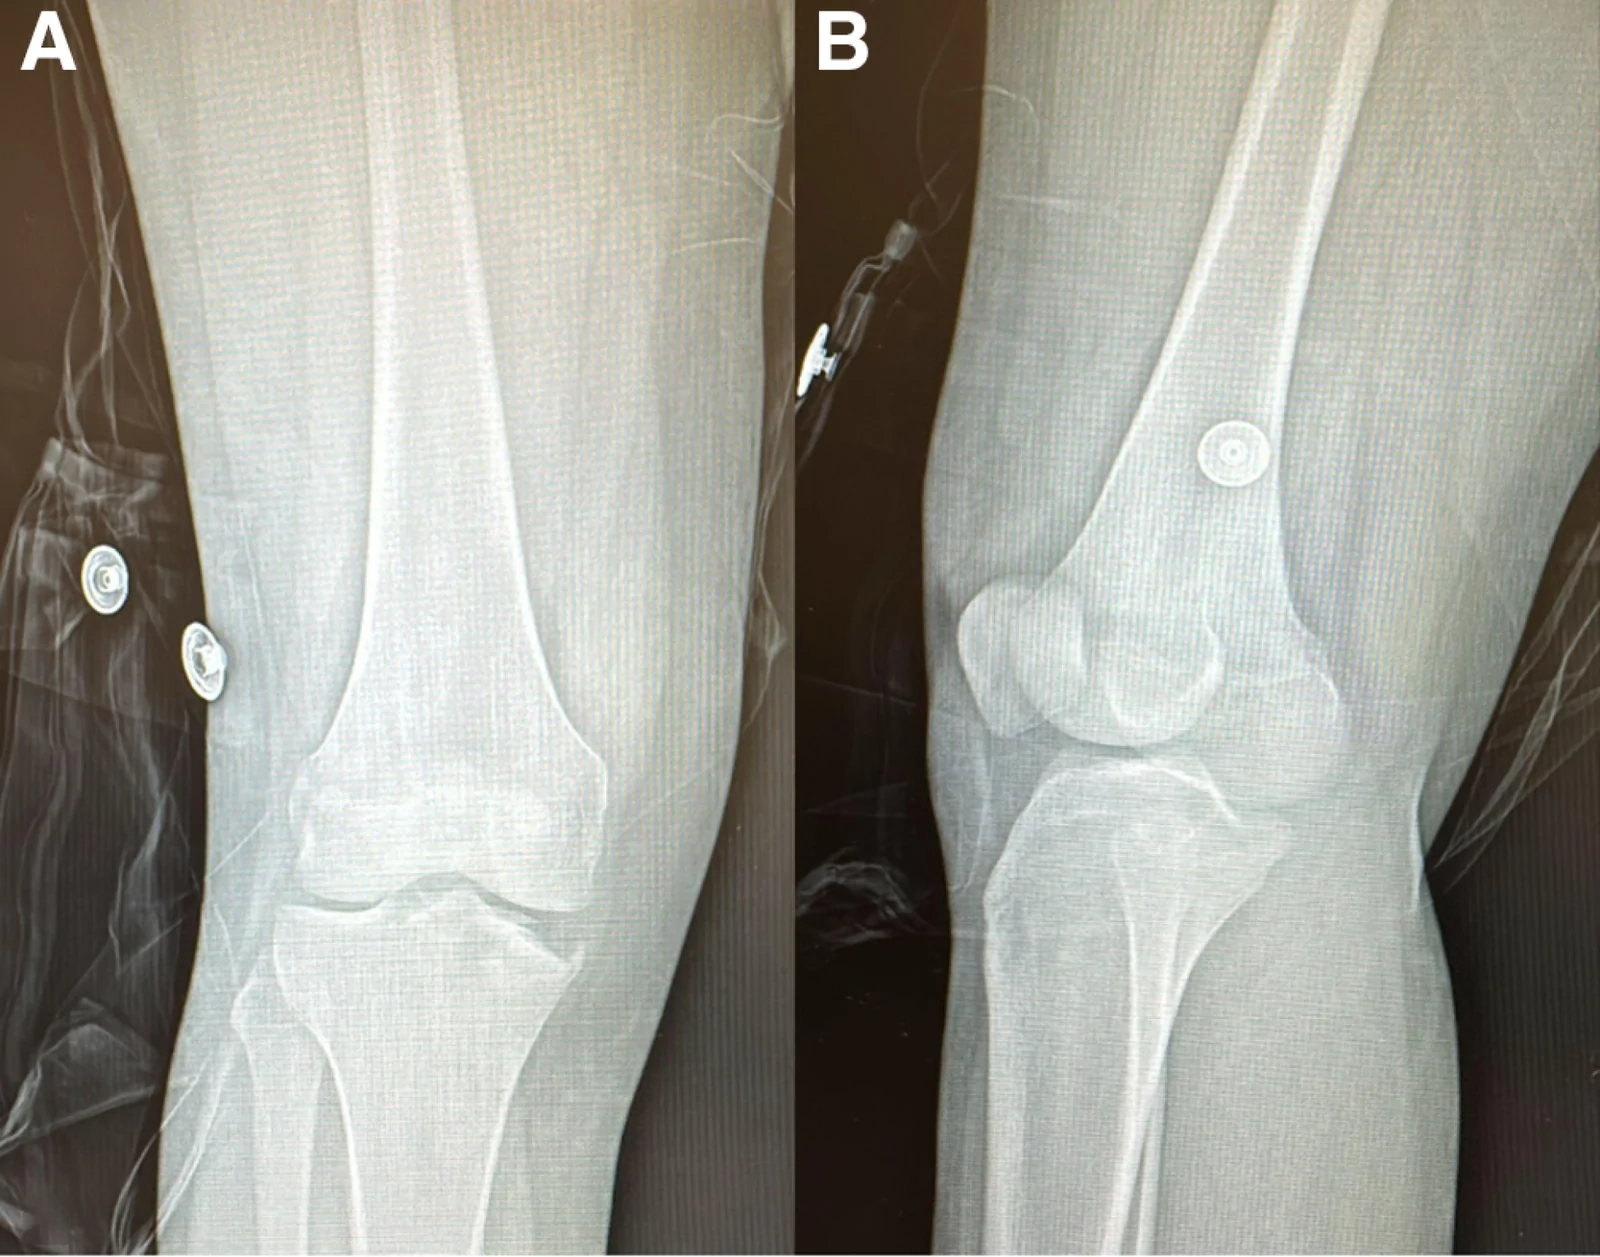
Preoperative radiographs. (A) AP radiograph demonstrating an isolated medial tibial plateau depression fracture; (B) lateral radiograph demonstrating depression of the medial tibial plateau without significant posterior displacement. AP = anteroposterior.
